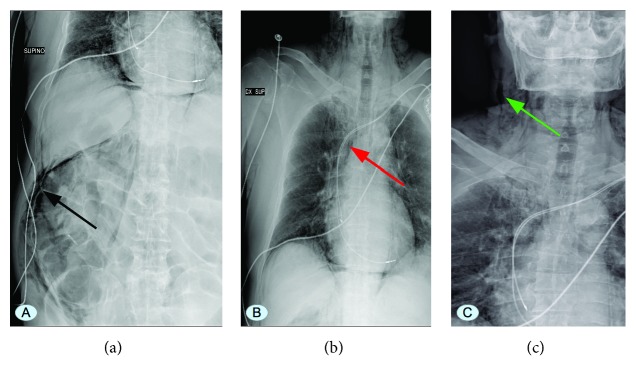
(a) Abdominal X-ray showing subcutaneous emphysema, pneumomediastinum, pneumoretroperitoneum, and right subdiaphragmatic free air (black arrow). (b) Chest X-ray showing pneumomediastinum (red arrow). (c) Neck X-ray showing right cervical subcutaneous emphysema (green arrow).
